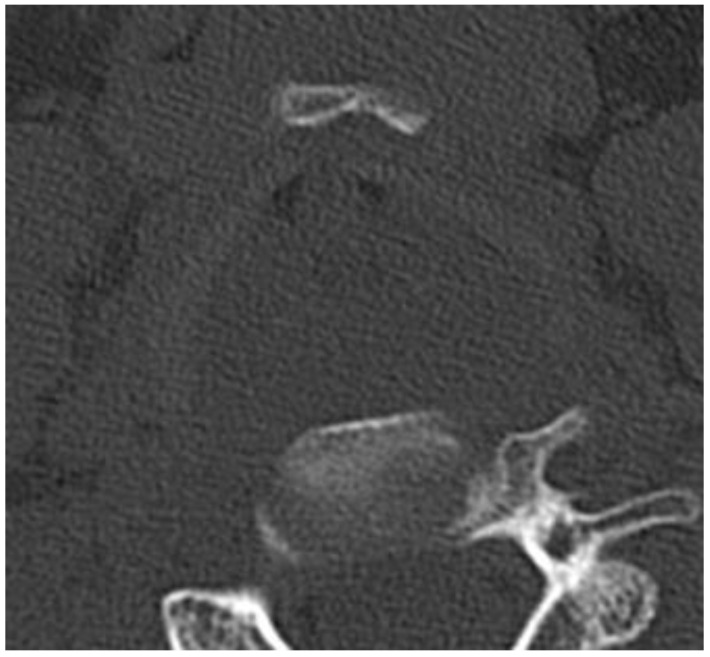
Axial CT section on the height of the thyroid cartilage. The cartilage in this sample is not calcified. Non-calcified structures posed a limitation in our study, since non-displaced fractures in these samples are not readily visualized.
